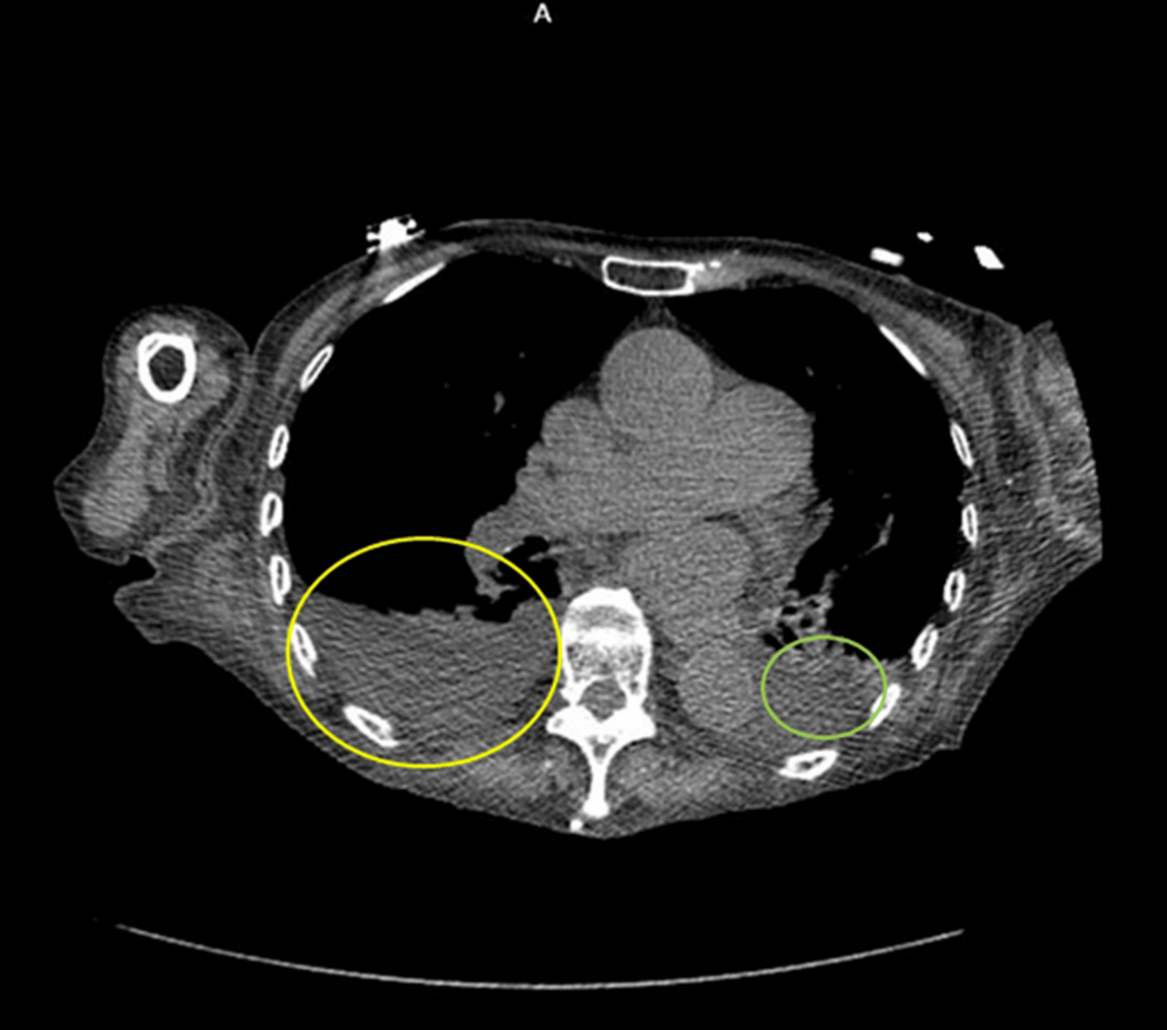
Axial chest CT without contrast. Small-to-moderate right (yellow) and small left (green) pleural effusions are seen, with no mediastinal calcification or lymphadenopathy.
